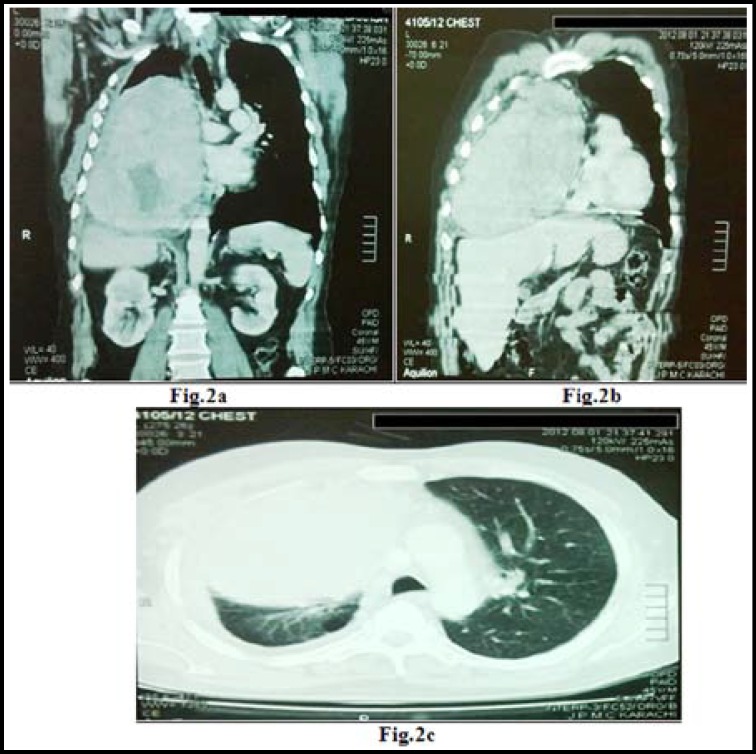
a, b, c: CT Scan of chest; shows a large heterogeneous mass with few sparks of calcification occupying almost whole of the right hemi thorax. Medially the mass is abutting and pushing superior vena cava, Aorta, right pulmonary artery and heart. Mass is also causing compression effects on the right hemi-diaphragm.
